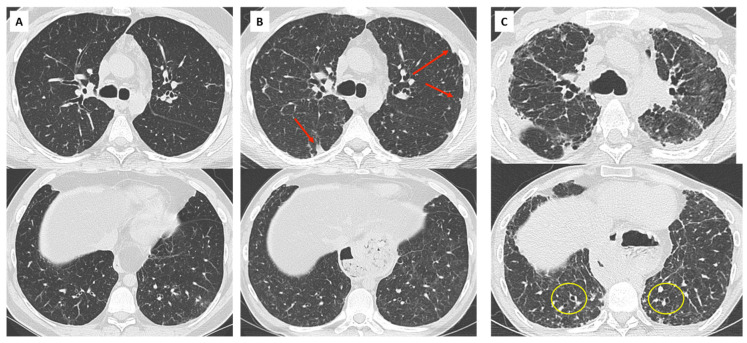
Mixed CLAD in a 30-year-old patient. (A) Diagnosis of CLAD in 2007 with initial obstructive phenotype. CT shows mild bronchial wall thickening; (B) progressive decline in TLC, suggesting an evolution towards a mixed CLAD (oct 2009). Note the apparition of subpleural opacities in the upper lobes (red arrows) and mild and diffuse ground glass opacities; (C) rapid functional and radiological deterioration, with upper predominant fibrosis and subpleural consolidation suggesting PPFE, mild cylindrical bronchiectasis in the lung bases (yellow circles).
